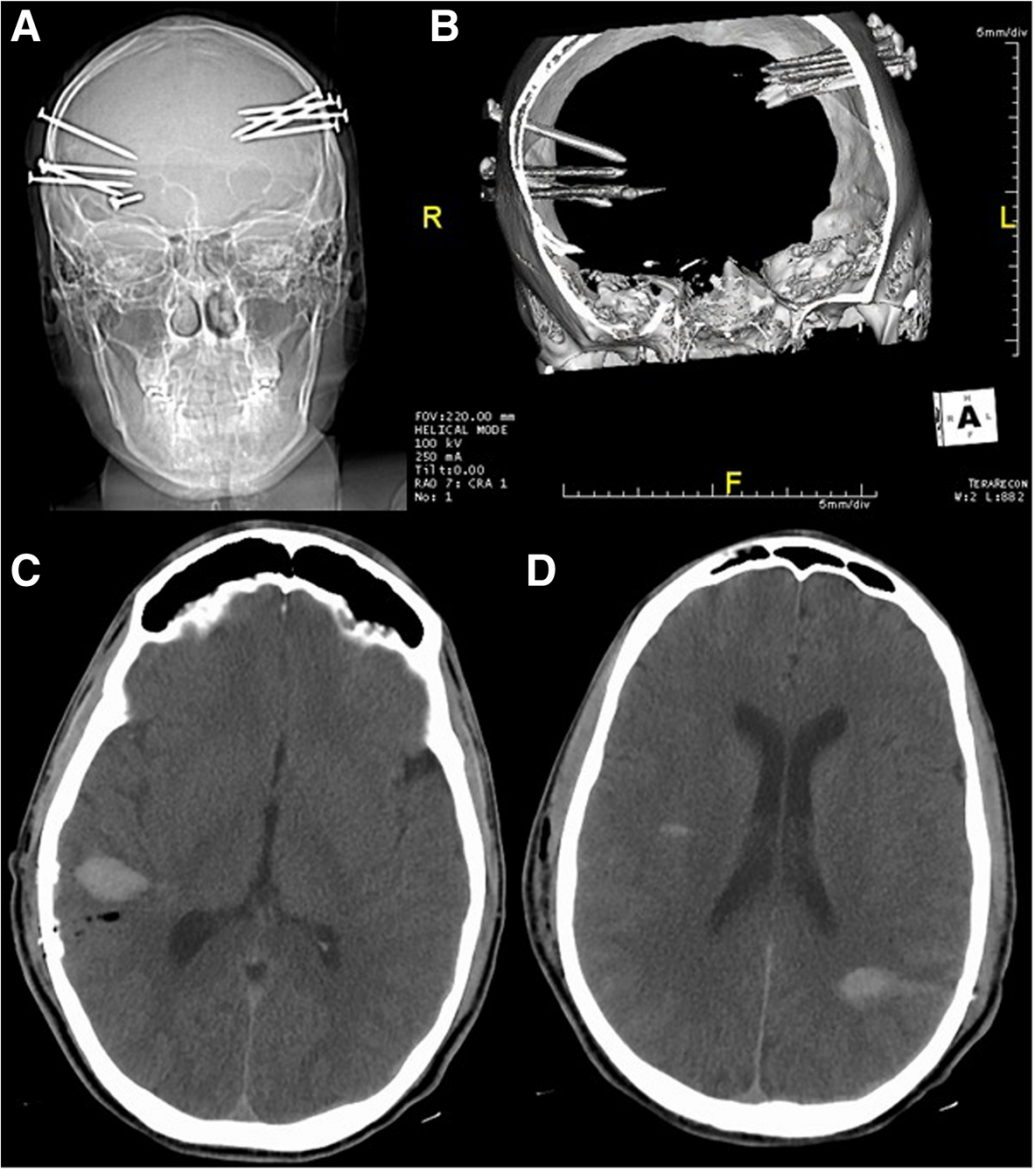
a Computed tomography scout. b Computed tomography three-dimensional reconstruction. There are ten nails projected over the temporo-parieto-occipital area bilaterally, five on each side, these caused significant artifacts on computed tomography and computed tomography angiogram (not shown here), but no major vessel injury was identified and there was evidence of parietal subarachnoid bleed. c, d Postoperative axial computed tomography. Multiple foci of intraparenchymal hemorrhage and associated subarachnoid hemorrhage seen in the left parietal and right frontal-temporal-parietal regions. Multiple tiny calcified bodies were now noted over the left parietal region; they probably represented small bone fragments introduced at the time of penetrating injury. These were obscured on the prior study due to metallic artifact
